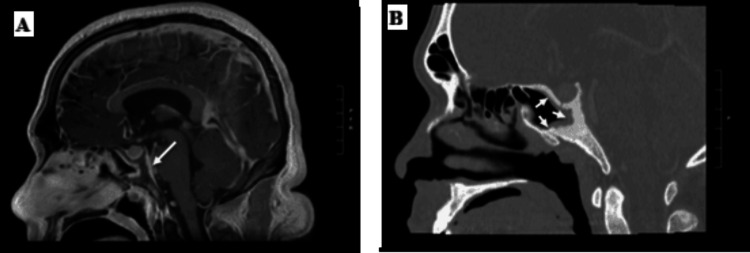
MRI and CT imaging of the clivus and surrounding sinuses (A) MRI sagittal T1 sequence with intravenous contrast shows abnormal marrow signal and enhancement of the clivus and dorsum sella (arrow). (B) CT of sinuses, sagittal view with bone window, shows thickening of the walls of the sphenoid sinuses (arrows) MRI: magnetic resonance imaging; CT: computed tomography
